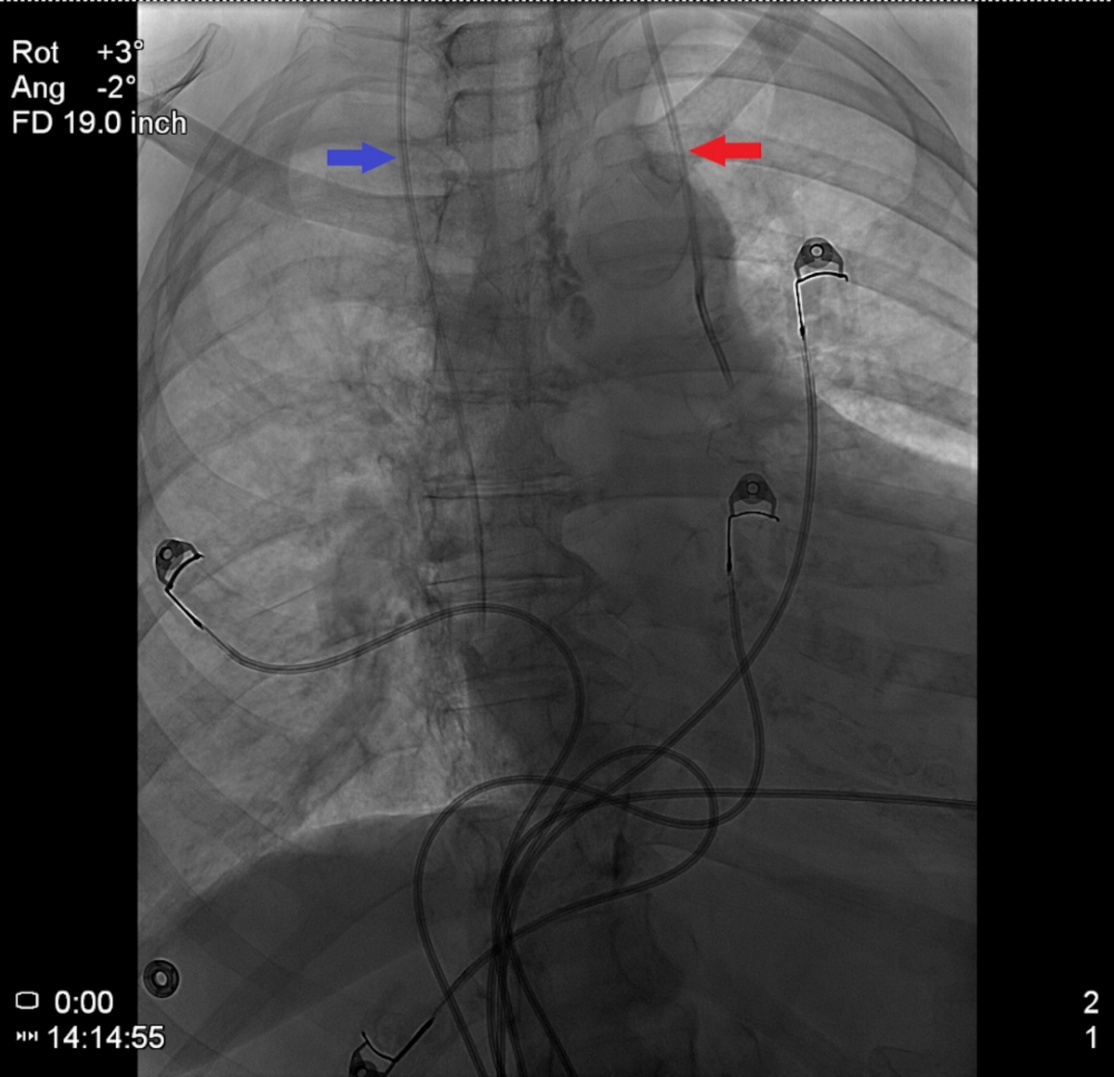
Chest radiograph with right-sided CVC (blue arrow) as well as the malpositioned left-sided catheter in the left IJV (red arrow), prior to removal of the latter. CVC, central venous catheter; IJV, internal jugular vein
